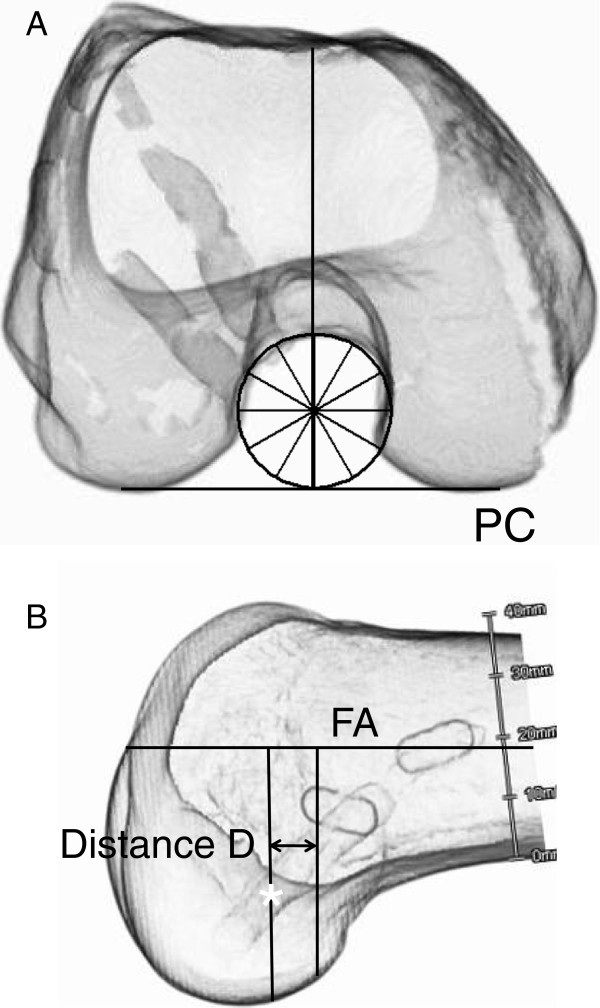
The T-3D CT images of the femoral notch in the axial view and the lateral view. On a photograph taken in the axial view (A) and the lateral view (B), the center of an actually created AM tunnel was measured by using the same 3-dimensional clock system as used in the first sub-study (PC and FA: See Figure 4).
